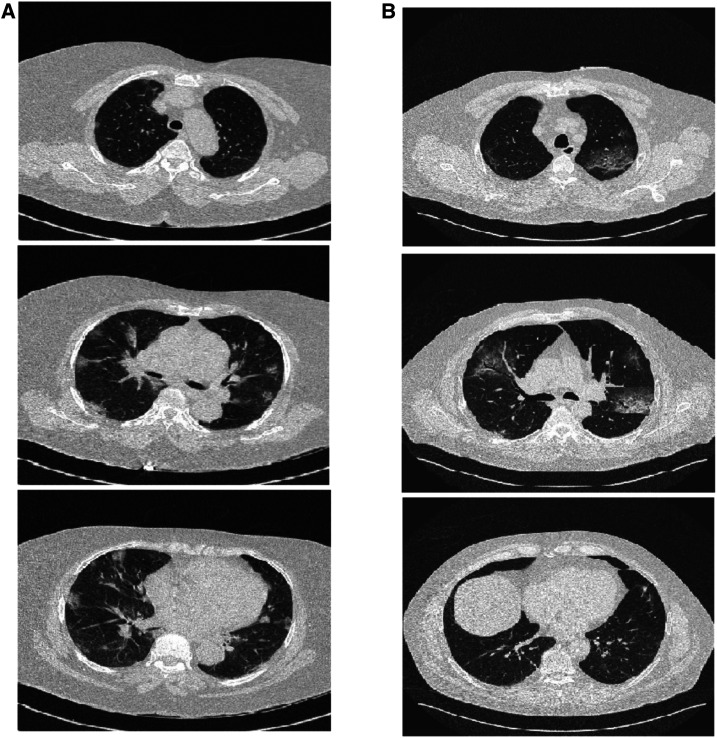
Chest computed tomography on the day of hospital admission for patients A (left) and B (right) at 10 and 12 days before intubation.
